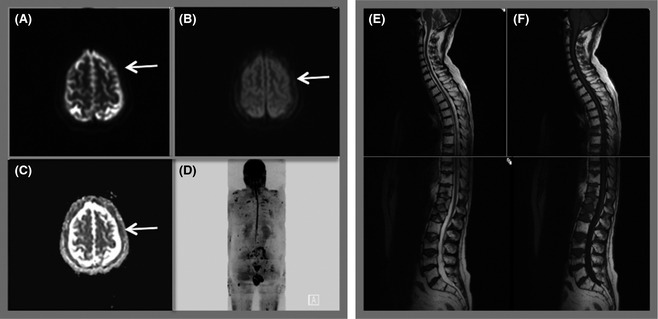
Whole body MRI protocol. Diffusion weighted magnetic resonance imaging (DW‐MRI) is performed axially using *b* values of 50 (A) and 900 (B). The system software automatically generates an apparent diffusion coefficient map (C) for each slice using the *b* 50 and 900 data. Axial images through the head demonstrate abnormal signal in the skull vault indicating infiltration (arrows). Radiographers post‐process the data to produce the 3‐dimensional inverse grey scale Maximum Intensity Projection image (d). Whole body DW‐MRI is supplemented by sagittal T2 (e) and T1 (f) weighted images of the spine. Total imaging time 45 min.
